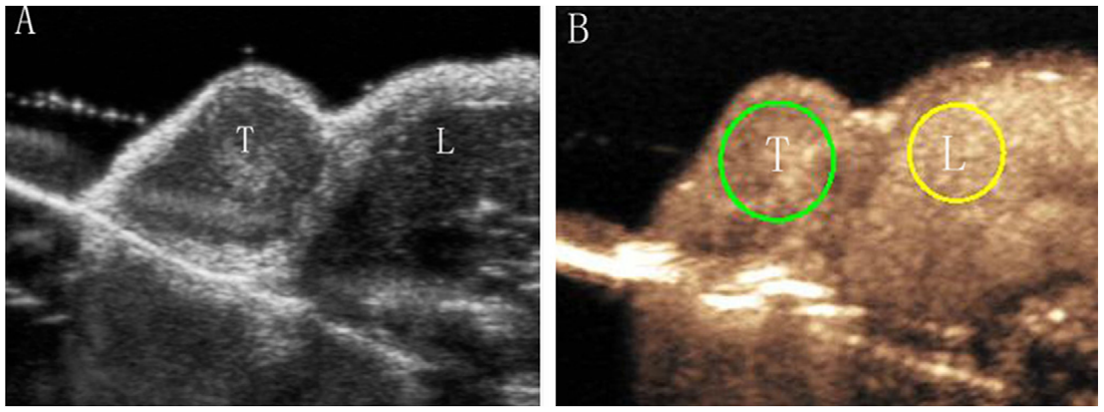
US image of a subcutaneous MCF7 breast cancer tumor. A, US scan showed gray-scale ultrasound image of tumor (T) adjacent to the right lobe of the liver (L). B, CEUS shows enhancement of normal liver parenchyma and tumor. Measurements obtained from one ROI drawn on the tumor (green ROI) and one drawn at matched depth in liver parenchyma (yellow ROI).
